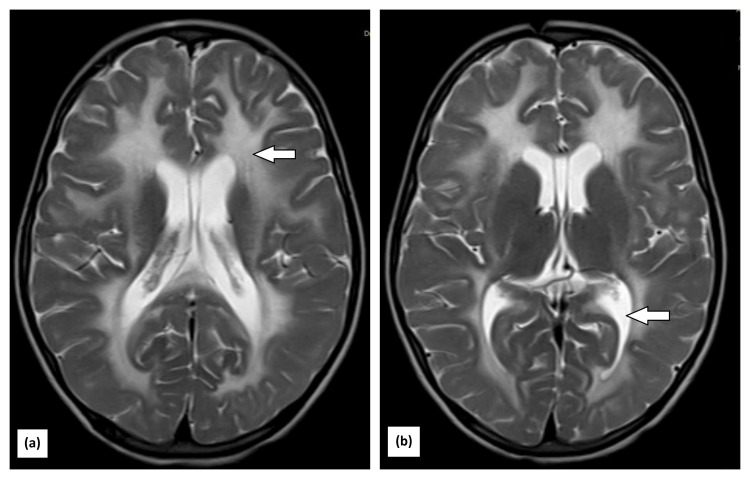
MRI showing (a) multiple ill-defined confluent T2 hyperintensities involving bilateral peri-ventricular white matter, deep white matter, and the bilateral fronto-parietal region. Tigroid appearance noted due to sparing of periventricular white matter; the bilateral external capsule and posterior limb of the internal capsule are involved; and subtle diffusion restriction in the splenium of the corpus callosum and along the lateral margins of white matter hyperintensity in both cerebral hemispheres; (b) bilateral cerebellar hemispheres involving white matter. All these findings support the diagnosis of vanishing white matter disease
